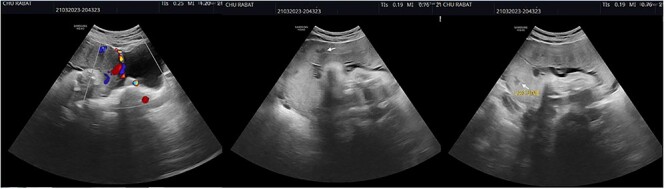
Ultrasound showing intra-placental lacunae (white arrows) as well as placental tissue protrusion beyond the uterine myometrium, as well as urinary bladder wall discontinuity and the extension of Doppler vascularity between serosa and the bladder (Fig. 1).
